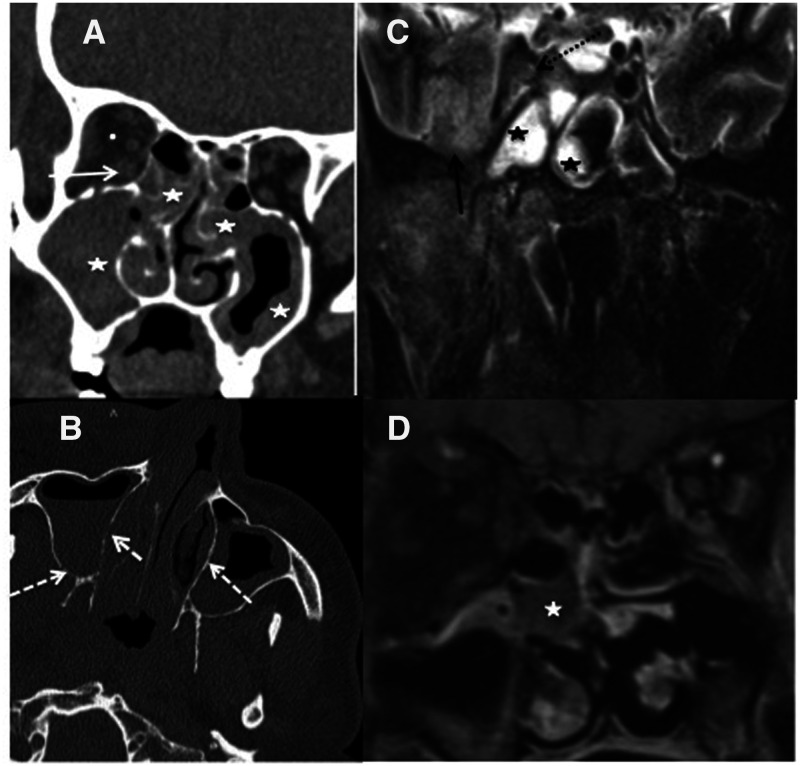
Radiological manifestations of proven cases of COVID-19–associated rhino-oculo-cerebral mucormycosis in different patients. (A) Computed tomography (CT) scan of coronal soft tissue window section showing mucosal thickening and soft tissue involving bilateral maxillary and ethmoid sinuses (white asterisks), fat stranding in the orbital fat (white dot), increase in bulk of extra ocular muscles with blurring of their margins (white arrow). (B) CT scan axial bony window section showing soft tissue in bilateral maxillary sinuses and right nasal cavity with presence of bony erosions in the walls of maxillary sinuses (white dashed arrows). (C) Magnetic resonance imagining (MRI) scan of coronal T2-weighted fat-saturated section showing soft tissue in sphenoid sinuses (black asterisks) with intracranial involvement shown by hyperintense signal in right temporal lobe (black arrow), ill-defined soft tissue in right cavernous sinus with occlusion of the right internal carotid artery (black dashed arrow). (D) MRI contrast-enhanced T1-weighted coronal section showing soft tissue thickening in right maxillary sinus with black turbinate sign (white asterisk).
